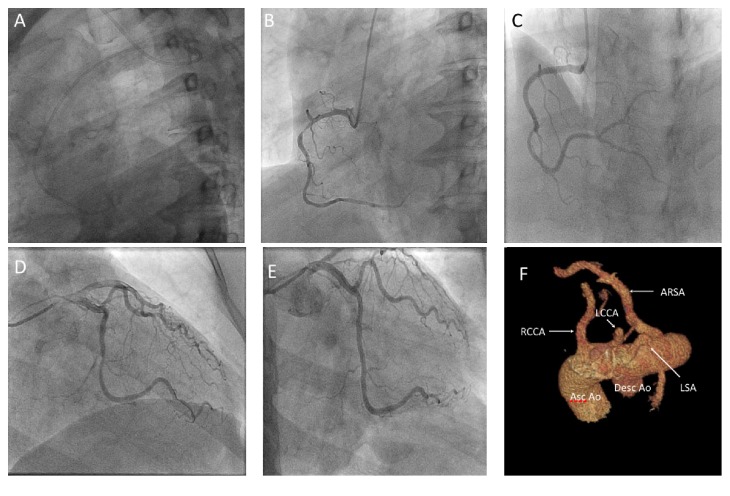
Angiographic images from Case  2: (A) lateral projection showing characteristic loop of ARSA; (B) left anterior oblique view of right coronary artery showing a 90% proximal lesion; (C) with subsequent stenting; (D) right anterior oblique caudal view of left coronaries with a 70% circumflex, 90% OM1, and 70% OM3; (E) final result; (F) A 3D volume rendered imaged from a contrast-enhanced CT angiographic dataset demonstrating vessels arising from the arch in the following order: RCCA, LCCA, LSA, and ARSA. ARSA, aberrant right subclavian artery; OM, obtuse marginal; RCCA, right common carotid artery; LCCA, left common carotid; LSA, left subclavian artery; Asc Ao, ascending aorta; Desc Ao, descending aorta.
